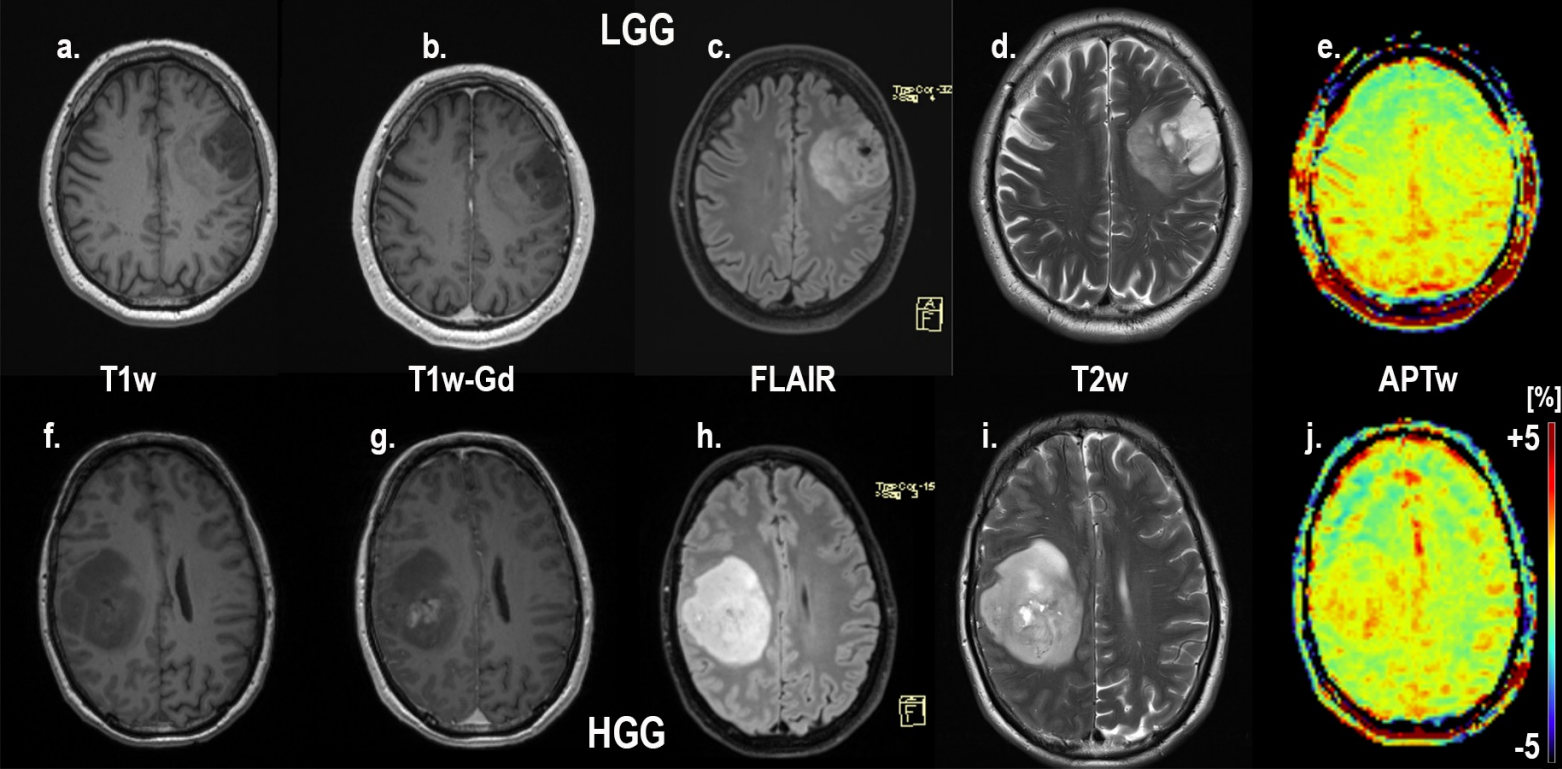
T1-MPRAGE without contrast, T1-MPRAGE with Gadolinium, FLAIR, T2w and APTw in subject 9 with a low grade glioma (a-e) and subject 10 with a high grade glioma (f-j). Of note is the increased APTw signal in the high grade glioma (j) compared to the low grade glioma (e). on APTw. Also notice the increased APTw signal in blood vessel regions of all patients. Subject 9 LGG (correctly diagnosed by all) and subject 10 HGG (misdiagnosed by the initial radiological classification as MET, correctly diagnosed by APTw).
